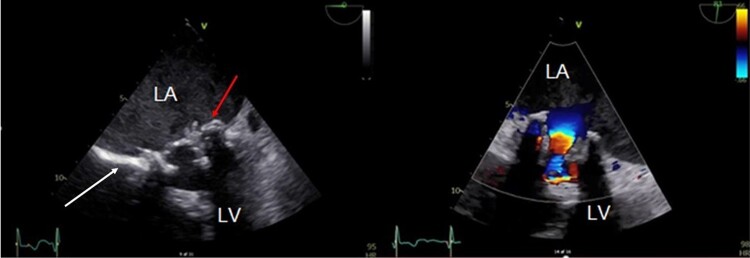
Transoesophageal echocardiography of Patient 1. The top arrow points at the mitral valve prosthesis and the vegetation. The lower arrow indicates the atrial septum. LV, left ventricle; RV, right ventricle.
